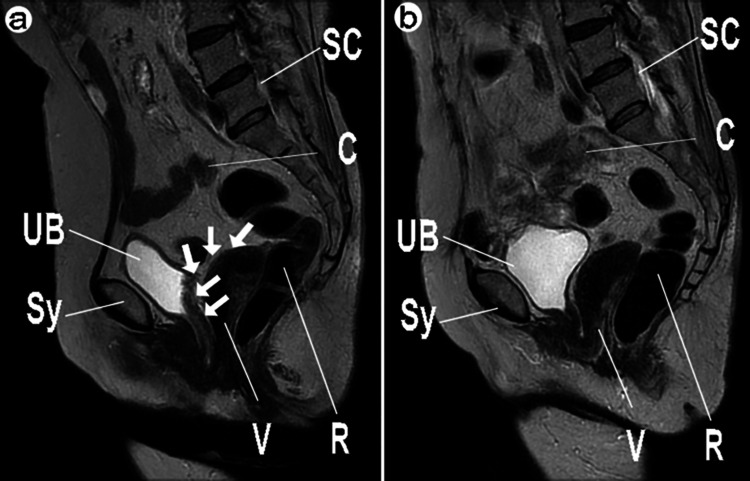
Pre- and Post-vNOTES MRI Imaging Showing Mesh Localization and Removal a: Before vNOTES procedure b: After mesh removal via vNOTES 1.5T MRI T2-weighted sagittal view, repetition time (TR) 4500 ms, flip angle 140 degrees, echo time (TE) 100 ms, slice thickness 3 mm, interval 3.5 mm, field of view (FOV) 32 x 36 cm, matrix 320 x 320 (Signa Creator, GE Healthcare, Chicago, USA). Arrows: Localization of the polypropylene mesh, notably clumped at the vaginal stump. UB: Urinary bladder; Sy: Symphysis pubis; SC: Spinal canal; C: Small intestine; R: Rectum; V: Vagina; vNOTES: vaginal natural orifice transluminal endoscopic surgery
